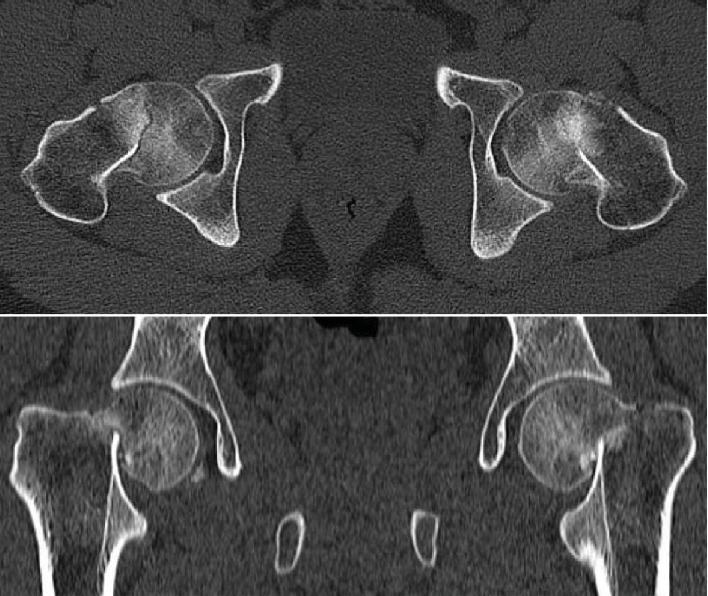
Axial and coronal pelvis CT scan cuts showing bilateral neck of femur fracture.
